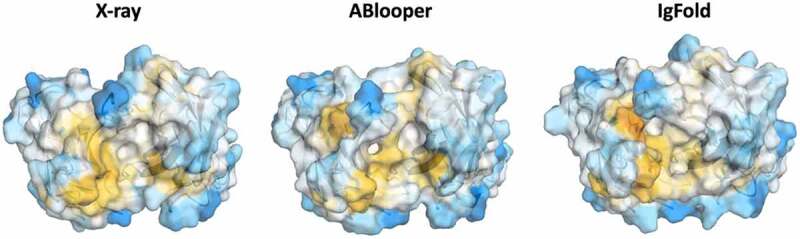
Surface hydrophobicity mapped on the X-ray structure and two antibody models. Surface hydrophobicity was assigned by the Whimley and White hydrophobicity scale. Hydrophobic areas are colored in yellow, while hydrophilic parts are depicted in blue.
